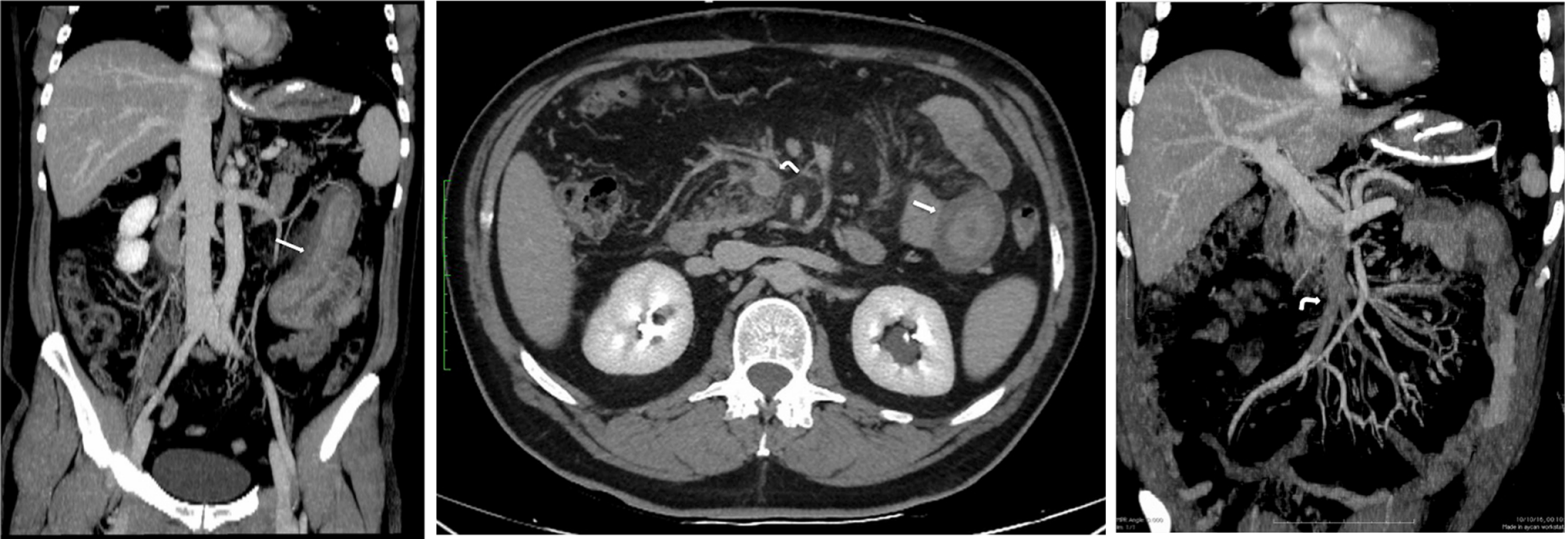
Preoperative contrast-enhanced CT scans of a 55-year-old male (patient No. 4) show thickening of jejunum loop (arrow) and extensive thrombosis of the SMV (curved arrow)
